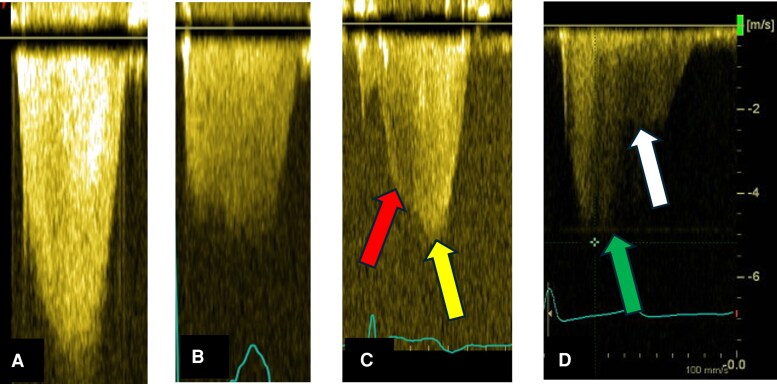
Pitfalls of spectral Doppler interrogation. (*A*) shows a well-aligned, blunted tricuspid regurgitation (TR) CWD signal in a patient with pulmonary arterial hypertension (right ventricular systolic pressure of 65 mmHg), whereas (*B*) shows a truncated TR CWD signal due to misalignment of the beam relative to the TR jet in the same patient; (*C*) shows a double TR CWD signal, due to mild prolapse of the tricuspid valve, creating two distinct parts of the CWD Doppler signal (red and yellow arrow); (*D*) shows a double ‘TR’ signal, which is due to contamination from an apical ventricular septal defect signal with left-to-right shunt (green arrow), creating a falsely high right ventricular pressure gradient of 107 mmHg. The white arrow indicates the TR CWD signal.
